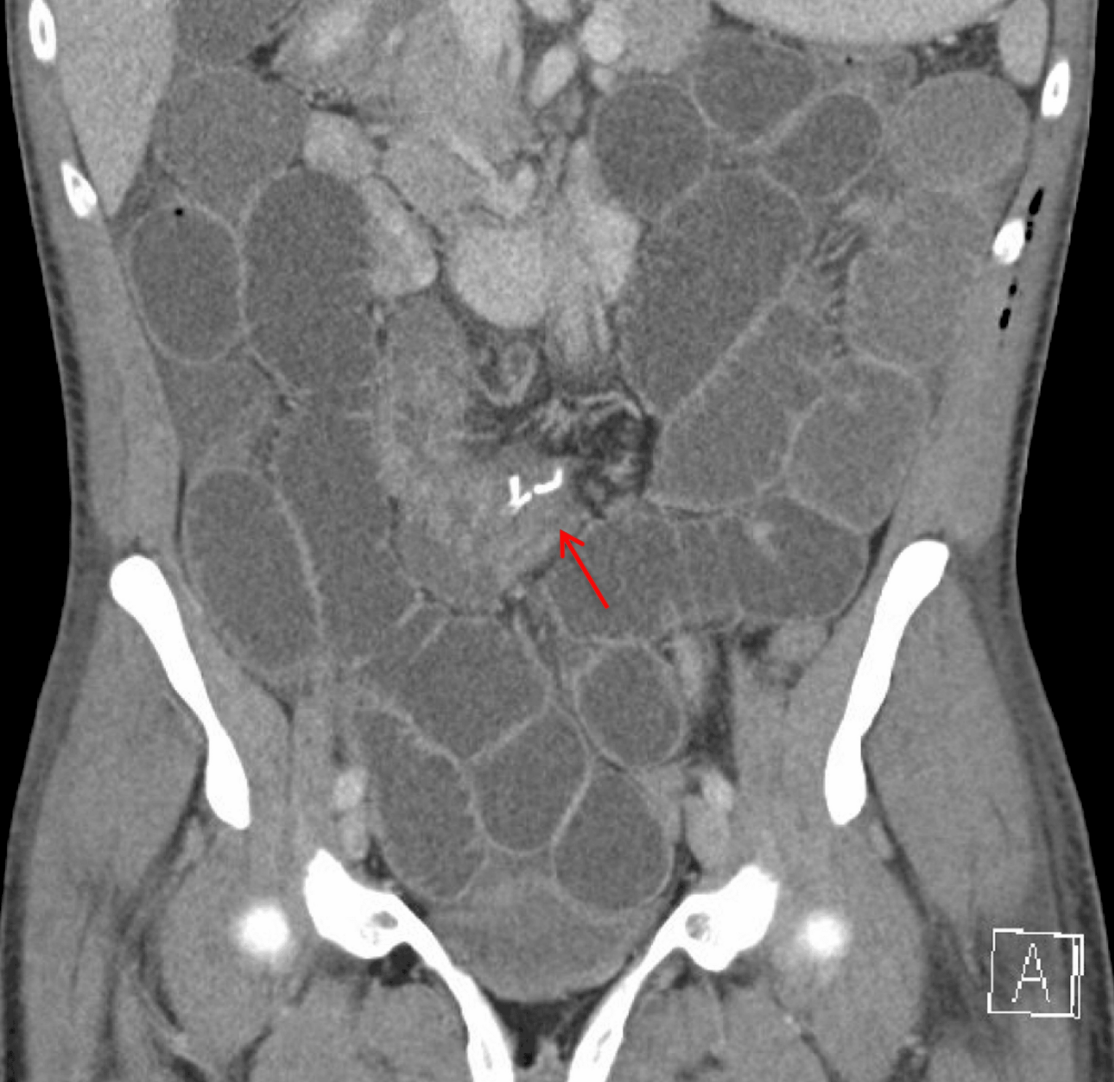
CT abdomen and pelvis with contrast (coronal view) shows diffuse dilated small bowel loops, a largely decompressed colon, and evidence of a transition zone near the anastomosis (red arrow). The transition zone represents the point where normal-caliber bowel transitions to dilated bowel, indicating the site of obstruction and serving as a critical finding in identifying the location of the underlying pathology.
